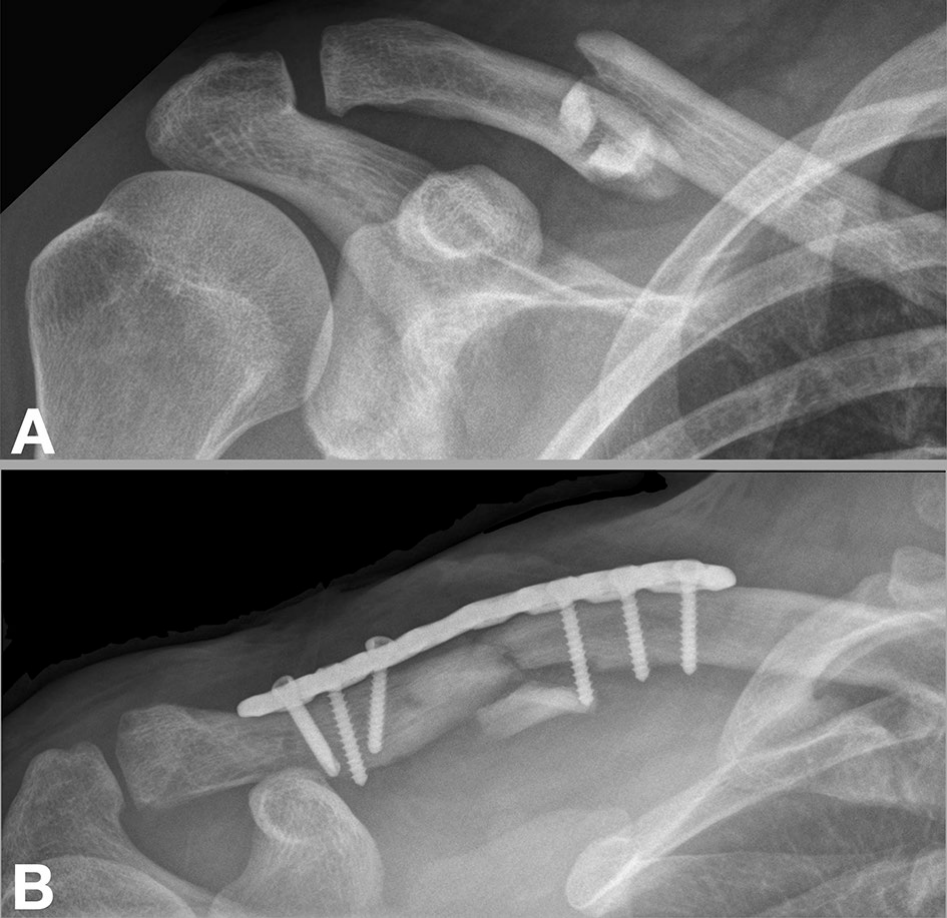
Radiographic anteroposterior images (right shoulder) of a 30-year-old female following a motorcycle accident demonstrating a midshaft clavicle fracture and fracture fixation with a superior plate. (A) Displaced midshaft clavicle fracture, (B) plate fixation with a superior plate.
